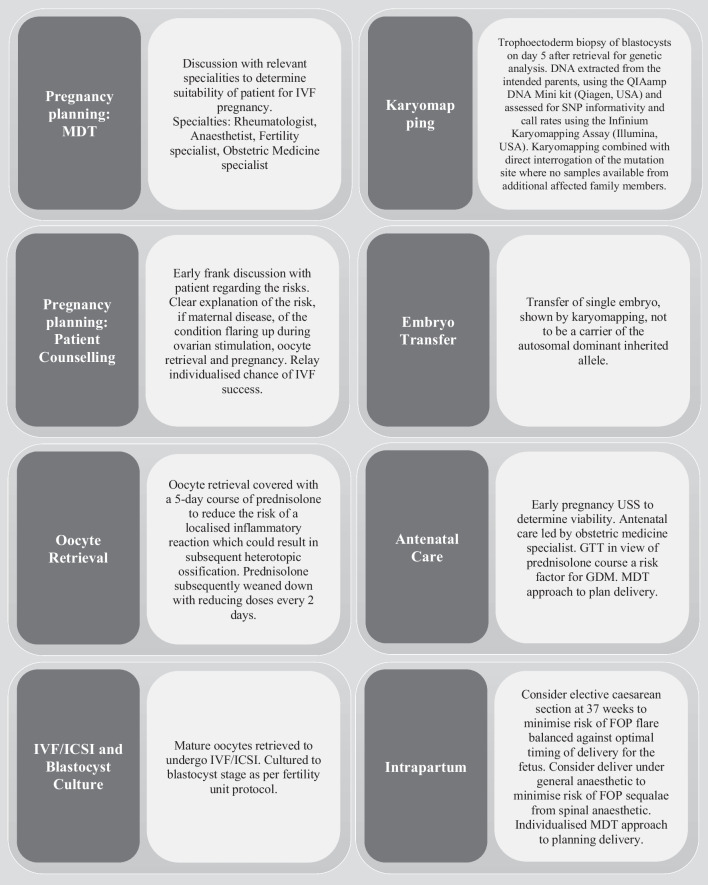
Guidance for specialists managing FOP patients during the preconception, antenatal and intrapartum periods. (Figure created by author S.M). *MDT* Multi-disciplinary Team, *IVF* In Vitro Fertilisation, *ICSI* Intracytoplasmic Sperm Injection, *SNP* Single Nucleotide Polypeptide, *USS* Ultrasound Scan, *GTT* Glucose Tolerance Test, *GDM* Gestational Diabetes, *FOP* Fibrodysplasia Ossificans Progressiva
